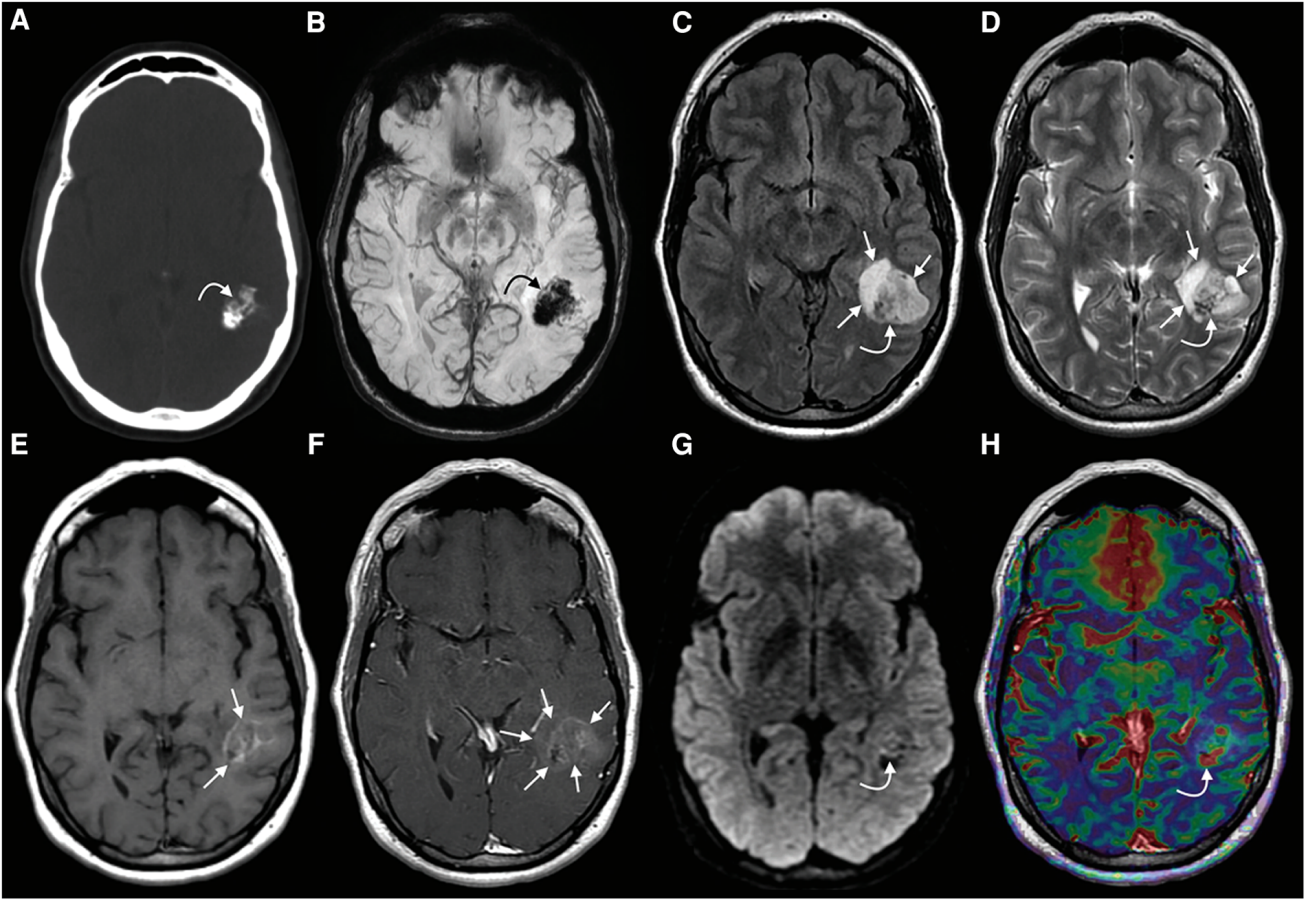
PLNTY. CT (A) image showing a mass within the left temporal lobe with dense intralesional calcifications, with a blooming signal on the corresponding SWI (B) (curved arrows in A and B). A heterogeneous signal is noted on both FLAIR (C) and T_2_WI (D) (curved arrows on C and D). A faint T1-hyperintense signal is noted in the central components of the tumor (straight arrows, E), while a greater extent of the mass demonstrates mild enhancement (straight arrows, F). A few tiny foci of mildly restricts diffusion were observed centrally (curved arrow, G). Some of the solid-appearing components demonstrates elevated relative CBV (curved arrow, H). This figure was originally posted by Benson et al. in *AJNR* (*American Journal of Neuroradiology*) in April 2020 (41).
